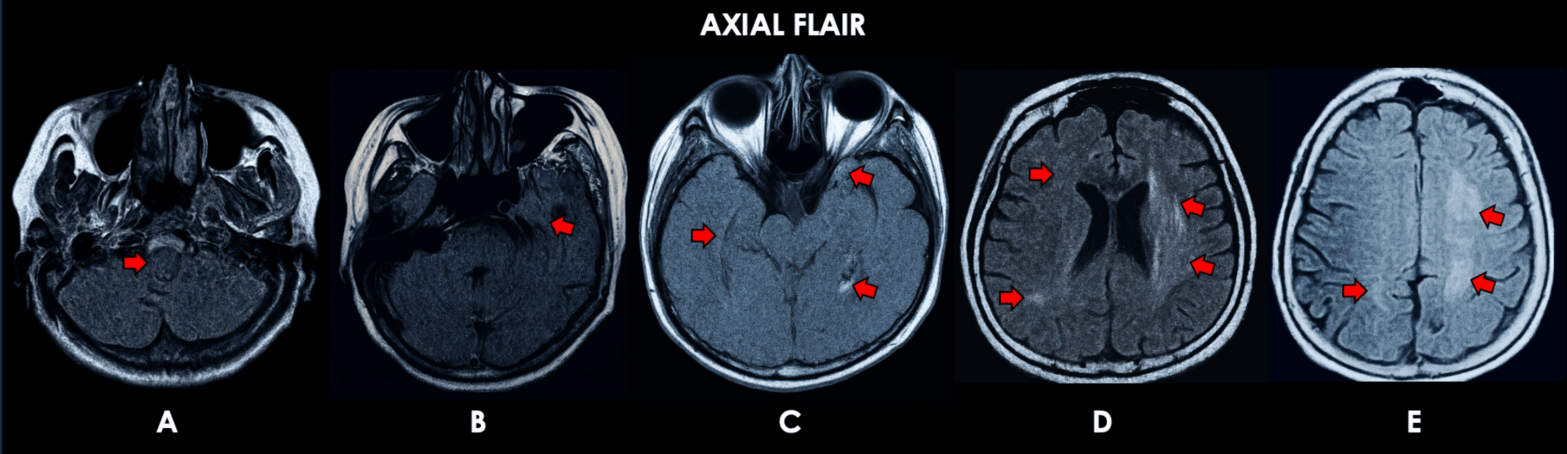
Representative cranial MRI sequences for patient #1. Cranial MRI obtained during the first hospital admission, approximately 2.5 months after the onset of illness. Representative axial brain MRI (FLAIR sequence) images show multiple FLAIR-hyperintense lesions in the right lateral medullary area (A); bilateral temporal and occipital areas (B, C); left frontoparietal area; right frontal and parietal areas; and the left corona radiata and left centrum semiovale (D, E). FLAIR: Fluid-attenuated inversion recovery.
